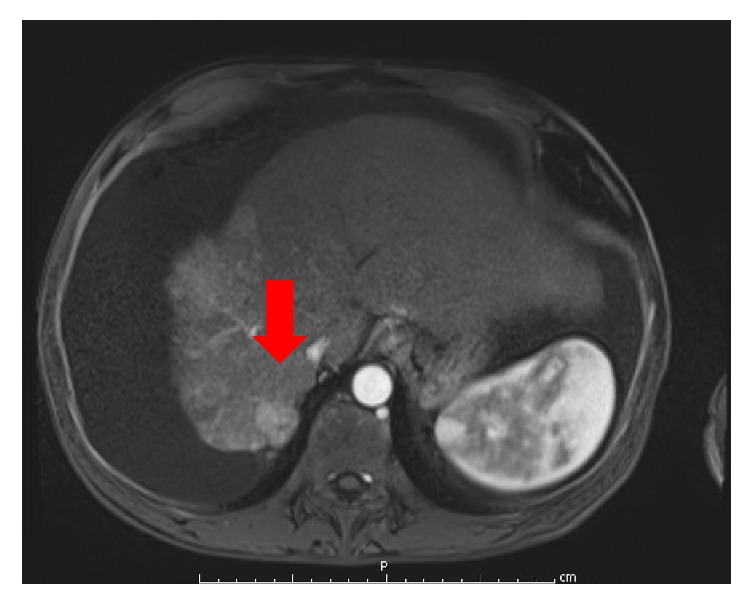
MRI image of an arterially enhanced lesion representing hepatocellular carcinoma seen with washout and delayed capsular enhancement. The tumor measures 2.2 cm in diameter and is located on segment 7 of the liver in close proximity to the tendinous portion of the right hemidiaphragm.
